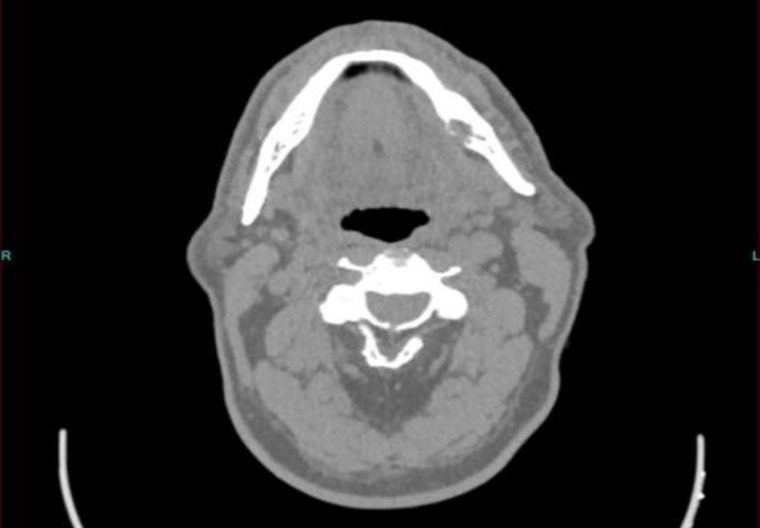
Axial view of CT images: a lytic lesion in the left mandibular corpus
